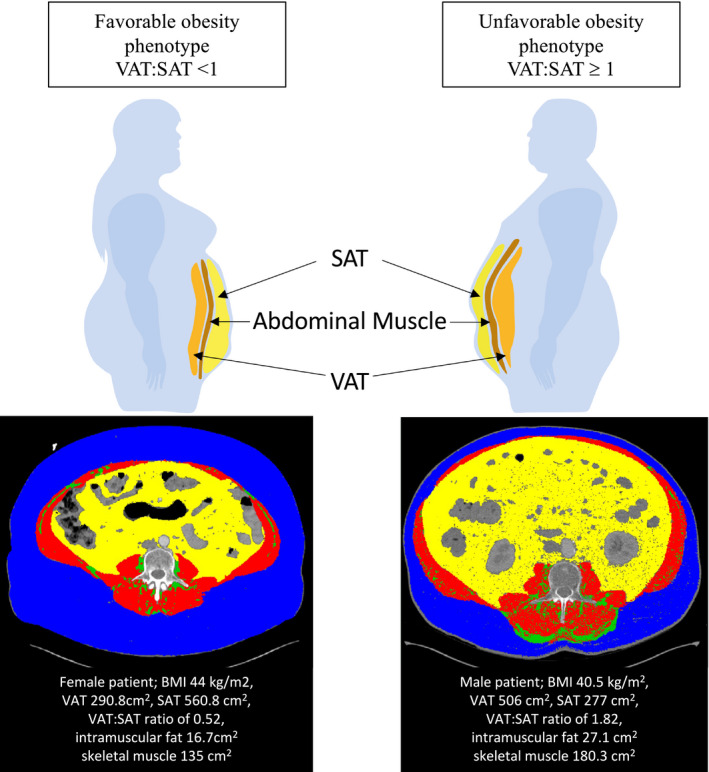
Favorable and unfavorable obesity phenotype. Abdominal adipose tissue distribution from a single computed tomography slice is shown with subcutaneous (blue) and visceral (yellow) adipose tissue, separated by the abdominal muscular layer (red). Intramuscular adipose tissue is seen in green. Favorable metabolic phenotype is seen in a female patient with a BMI of 44 kg/m^2^ and a visceral to subcutaneous adipose tissue ratio (VAT:SAT ratio) of 0.52. Unfavorable metabolic phenotype is seen in a male patient with a BMI of 40.5 kg/m^2^ and a VAT:SAT ratio of 1.82. BMI indicates body mass index; SAT, subcutaneous adipose tissue; and VAT, visceral adipose tissue.
